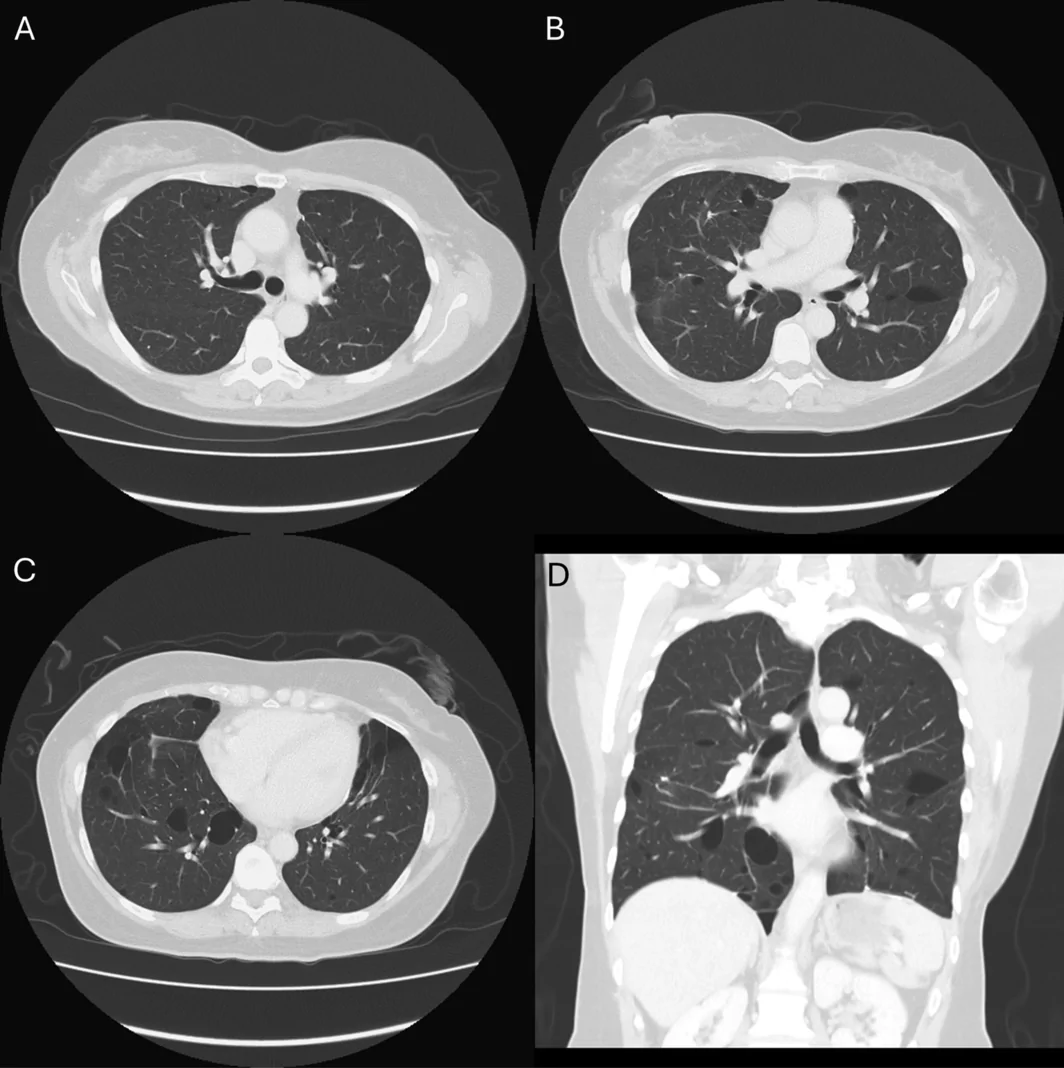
Axial chest CT images at different levels of the chest (A, upper; B, middle; C, lower) and coronal view (D) show multiple thin‐walled bilateral pulmonary cysts with medial and lower‐lobe predominance.
